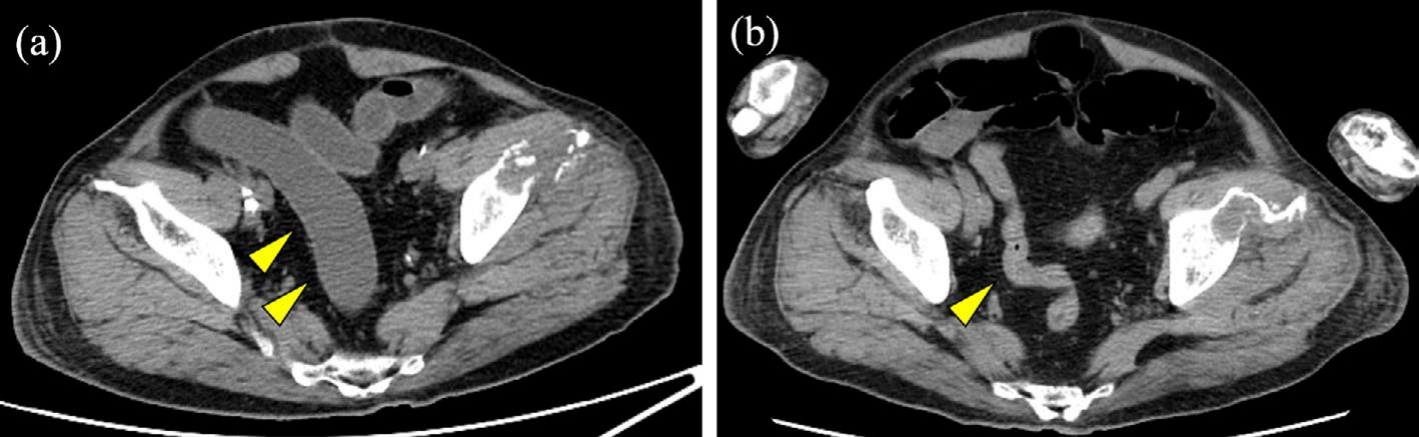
(a) Abdominal CT scan on admission (Day 17) showing edematous intestinal mucosa, dilation, and fluid accumulation inside in the sigmoid colon and rectum. (b) Abdominal CT scan on Day 25 revealing mucosal edema and obstruction extending from the sigmoid colon to the rectum.
